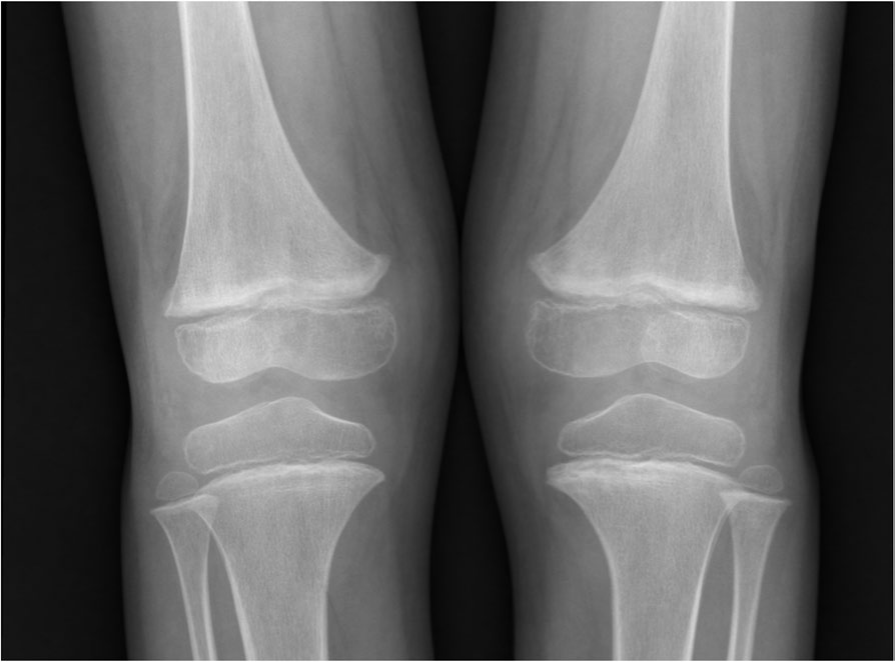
Both knees x-ray showed metaphyseal fraying and hypolucent lesions at metaphyses of both femurs
